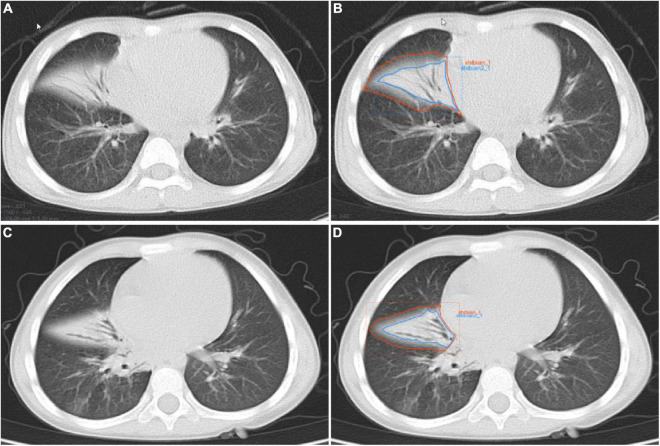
Manual delineation on lung window CT images in a 25-month female patient with mycoplasma pneumoniae pneumonia (A,B) and a 21-month male patient with streptococcus pneumoniae pneumonia (C,D). CT shows the similar appearances with consolidation and surrounding halo in middle lobe of right lung (A,C), and two ROIs (blue line and orange line) are delineated in each patient.
